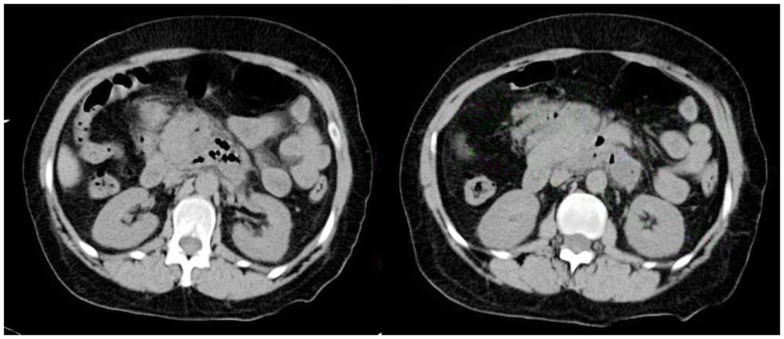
CT of the abdomen shows an abnormal, poorly defined collection (6.9 cm × 4.7 cm) of gas and inflammatory changes in the region of the head and uncinate process of the pancreas and dorsal and inferior to the body of the pancreas. A more focal (2.1 cm × 1.9 cm) fluid density along the posterior left aspect is noted.
